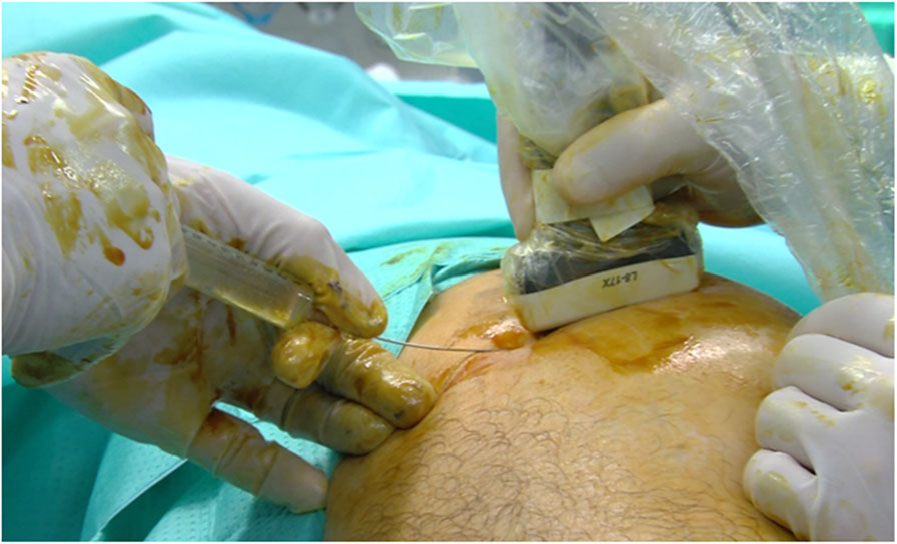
Ultrasound-guided insertion of the guide needle
